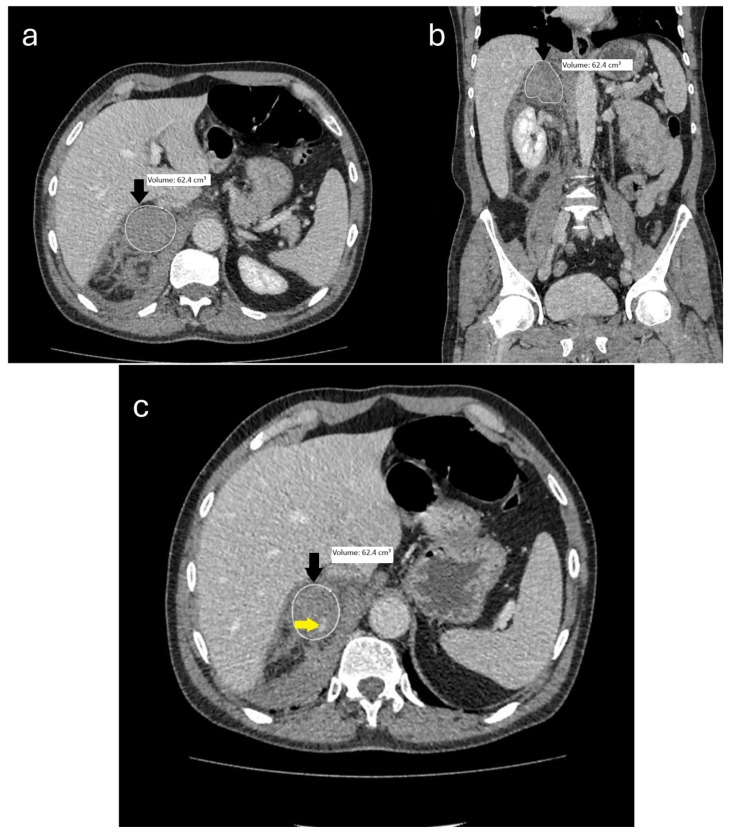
A 63-year-old male patient was admitted to the emergency department following a motor vehicle accident. Contrast-enhanced axial (a,c) and coronal (b) abdominal CT images demonstrate a hematoma in the right adrenal gland (black arrows), with associated hemorrhagic fluid extending into the right periadrenal and perirenal spaces, as well as Morrison’s pouch. On the portal venous phase CT (c), the yellow arrow indicates contrast extravasation within the adrenal hematoma, suggestive of active bleeding.
